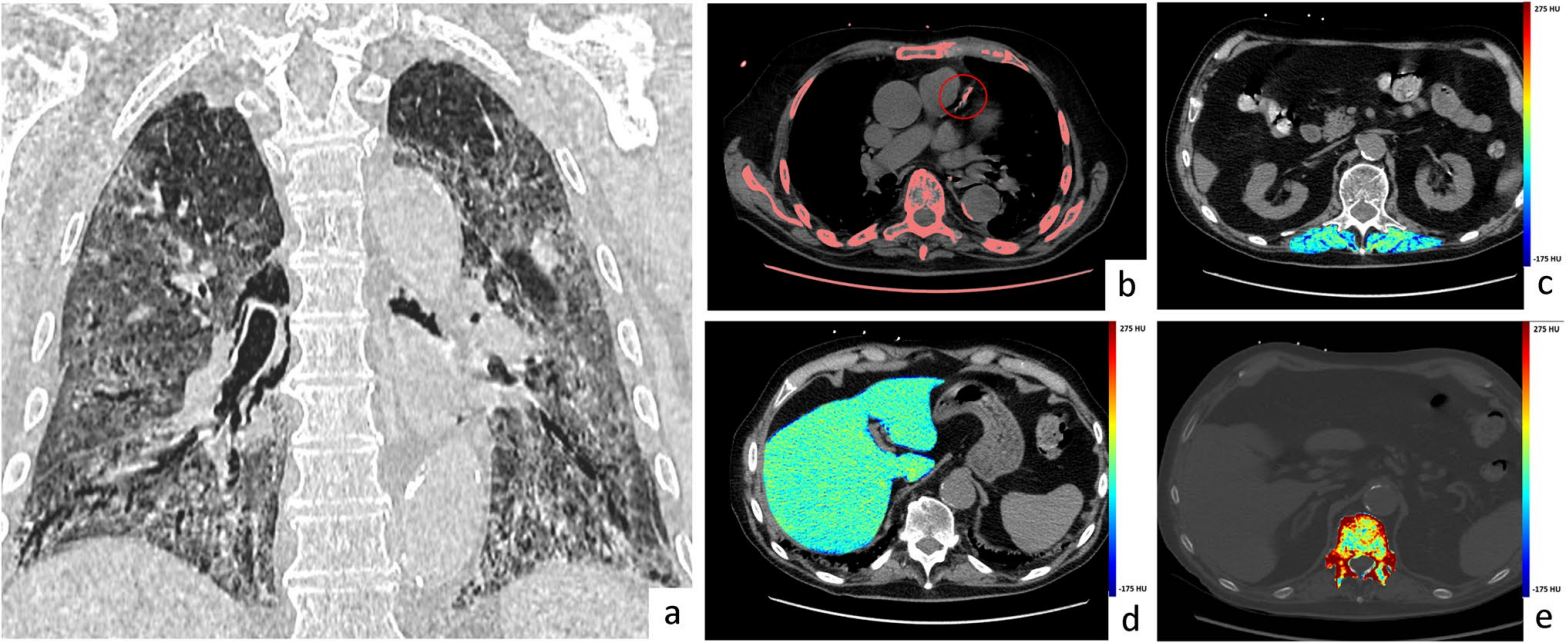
Exemplifying case of admitting chest CT findings in non-survivor. Male patient, 84 y.o., with a clinical history of hypertension, diabetes, and chronic kidney disease. At the hospital admission, vital signs were stable but SaO2 in ambient air was 87%. Laboratory data were collected, in particular: WBC 13,000/mm^3^, C-reactive protein 1.18 mg/dL. CT scan was performed the same day of admission, revealing an important involvement of the lung parenchyma (Pneumonia score 4, a) along with a moderate coronary artery calcium score (Agatston score 207, b), a reduced attenuation of paravertebral muscles, indicating the presence of myosteatosis (mean attenuation 27.5 HU, c), a liver attenuation suggestive for steatosis (mean density of right and left lobe 40 HU, d), indicating the absence of steatosis, and reduced trabecular attenuation of D12 vertebral bone (94 HU, e). The patient was admitted to Intensive Care Unit and after 27 days of hospitalization died
